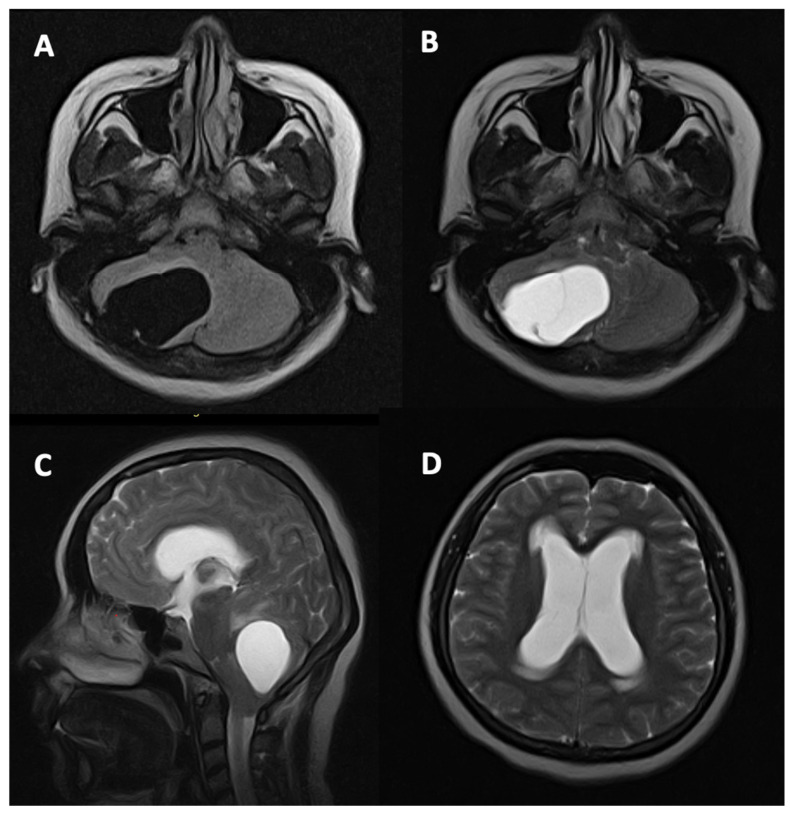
Preoperative MRI of the brain. Axial T1WI (A), axial T2WI (B), sagittal T2WI (C), and axial T2WI (D) demonstrated a well-circumscribed cystic lesion in the right cerebellar hemisphere measuring 5.61 × 4.24 × 3.45 cm, causing a significant mass effect with compression of the brainstem and fourth ventricle. Secondary obstructive triventricular hydrocephalus was present, with dilatation of the lateral and third ventricles and periventricular hyperintense signal changes on T2WI, consistent with transependymal cerebrospinal fluid flow (Evans Index—0.42). Inferior displacement of the cerebellar tonsils was observed, indicating elevated intracranial pressure.
